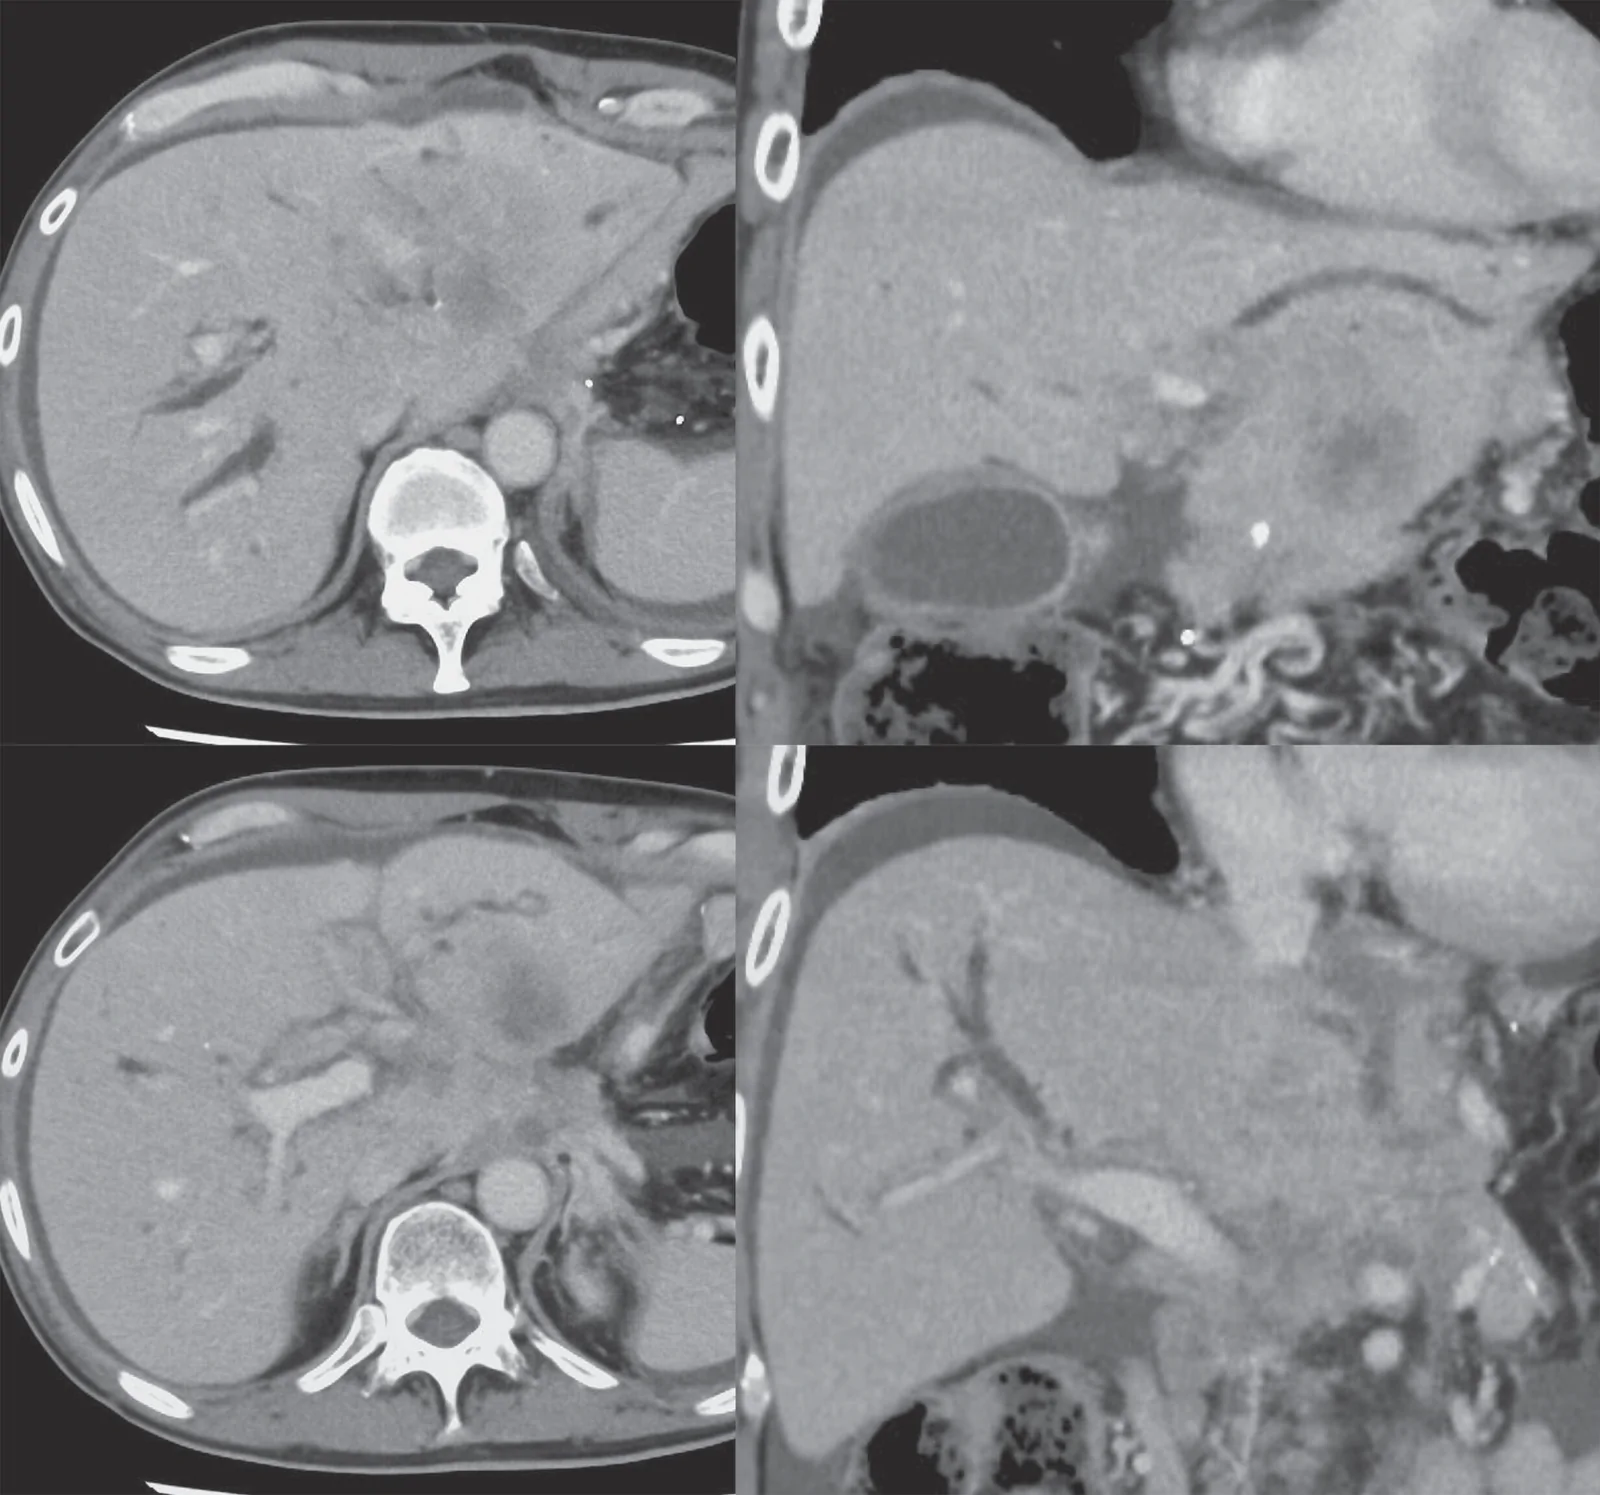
Abdominal contrast-enhanced computed tomography (delayed phase)
